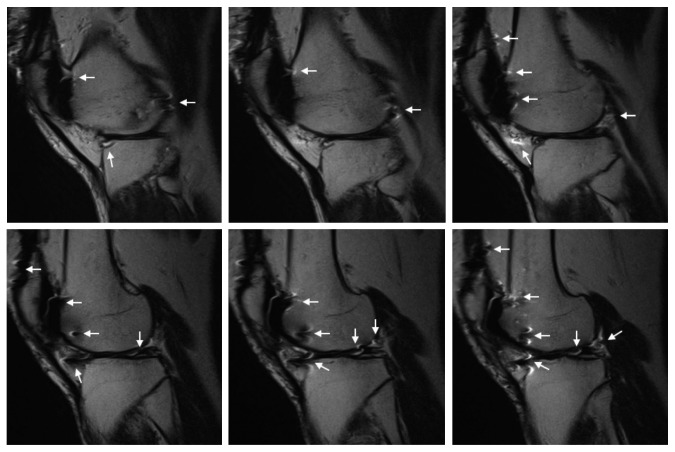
Sequential sagittal T2-weighted metal reduction MR images of the right knee lateral compartment. The presence of metal artifacts (white arrows) obscures accurate evaluation of the lateral meniscus.
